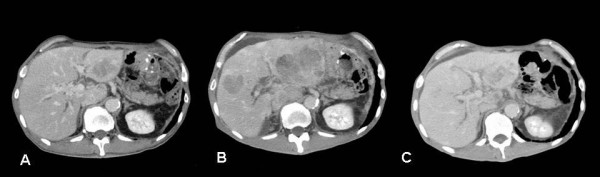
(A) Enhanced CT revealed that liver metastases had decreased in size following initial therapy. (B) After 5 months of treatment, multiple liver metastases reappeared in segments 1, 3, and 5. (C) Following second-line treatment with 5-FU/paclitaxel and paclitaxel monotherapy, liver metastases either regressed or disappeared.
